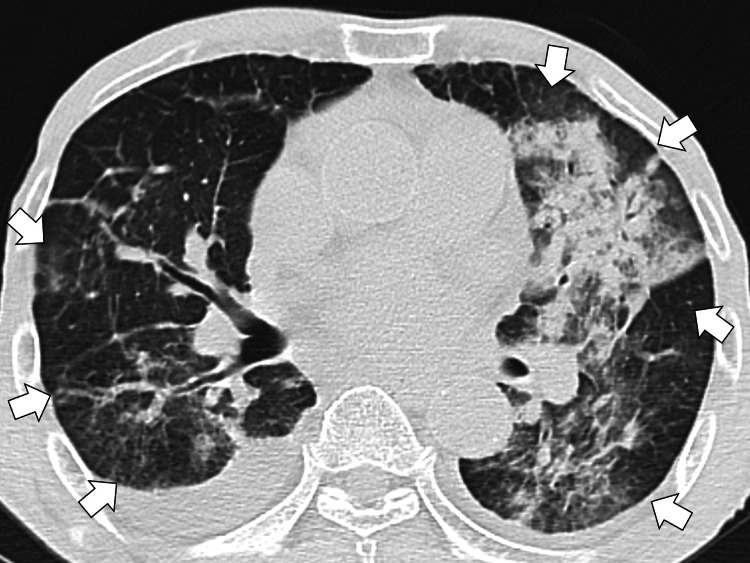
Chest computed tomography further confirming sputum impaction and infiltration on both lungs (white arrows)
